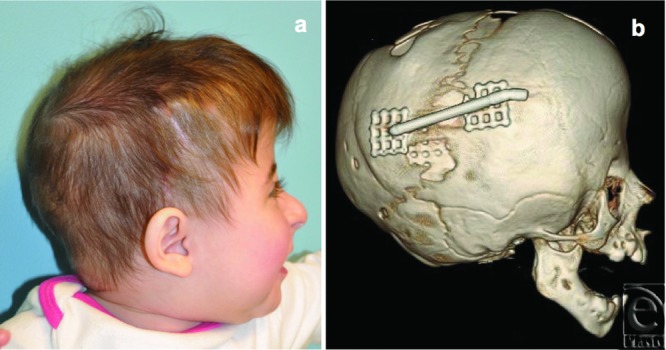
Clinical (*a*) and radiographic appearance (*b*) after 3 months of bony consolidation. Distraction hardware was subsequently removed.
